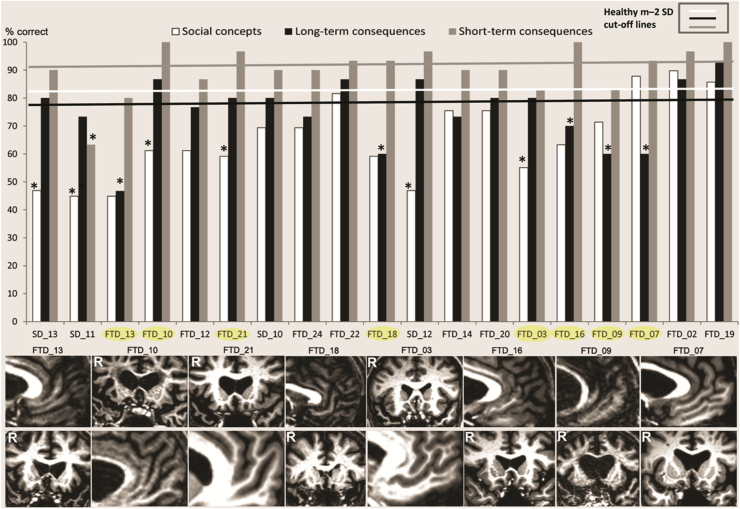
Performance on the Social Concept (white), Long-term Consequences (black), and Short-term Consequences (grey) task conditions are displayed for each patient (% correct). Patients are ordered according to their Addenbrooke's Cognitive Examination-R scores (left for lowest to right for highest scores). White, black, and grey lines represent healthy control means – 2 standard deviation cut-off values for each task. Using a well-established method (Crawford & Garthwaite, 2005), significant dissociations at *p* < .0026 (corresponding to an approximate Bonferroni-corrected *p* < .05 across 19 single patient cases) for Long-term versus Short-term Consequences and Social Concept versus Long-term Consequences conditions were marked with an asterisk. There was one patient with a selective impairment on Long-term Consequences versus Social Concepts (FTD_7). Another patient displayed a selective impairment on Short-term versus Long-term Consequences (SD_11). The upper row of T1-weighted MRI scans displays coronal sections through the anterior temporal lobes for 3 patients showing a Social Concept-selective impairment relative to Long-term Consequences (FTD_10, FTD_21, FTD_3), no MRI scans were available for additional 3 patients showing this dissociation (SD_13, SD_11, SD_12). The upper row also shows mid-sagittal slices through the frontopolar and ventromedial frontal cortices of all the 5 patients exhibiting a selective impairment for Long-term versus Short-term Consequences (FTD_13, FTD_18, FTD_16, FTD_09, FTD_07). The lower row displays slices to depict the 2nd region of interest for comparison (frontopolar for patients with Social Concept-selective and anterior temporal for patients with Long-term Consequences-selective impairments).
